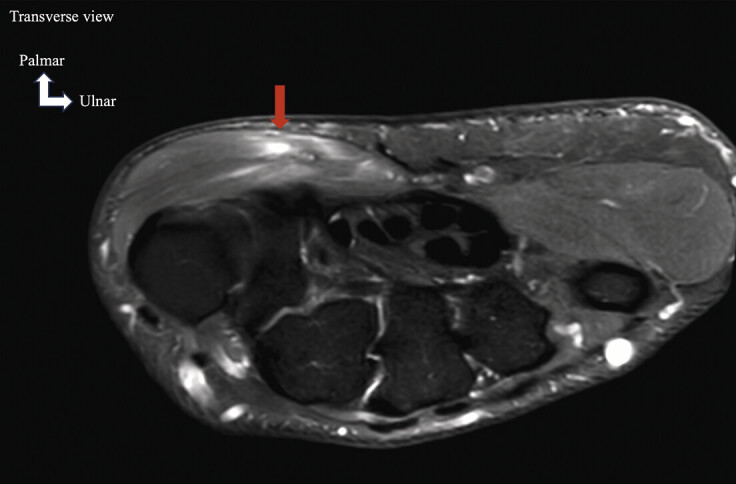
Contusion of the thenar muscles during direct trauma. Hand pain following a fall with landing on the thenar eminence. Suspected scaphoid fracture. Axial FS PD MRI shows a thenar muscle injury with significant edema (red arrow).
